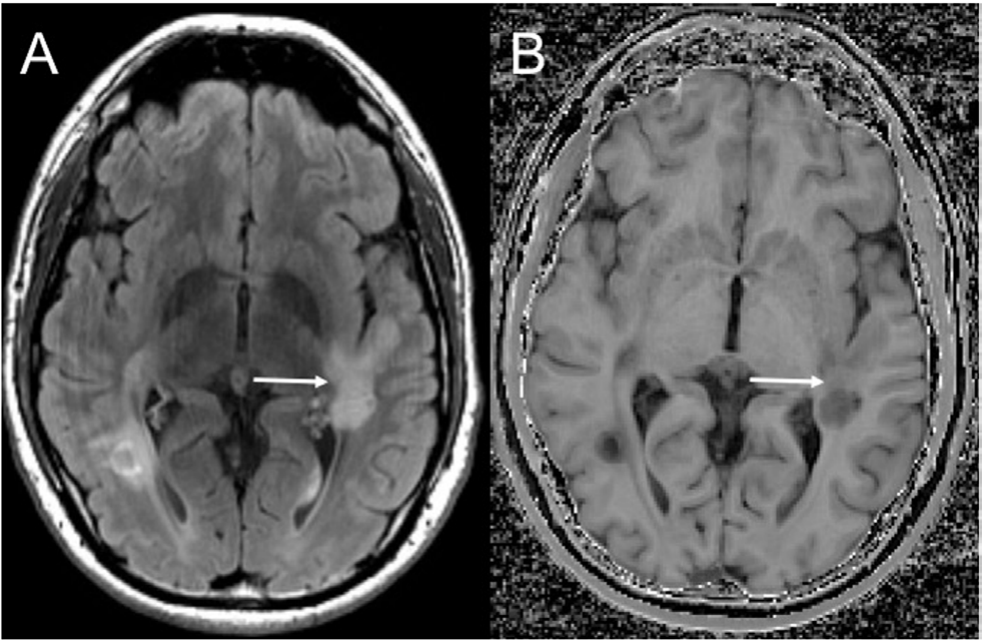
Magnetization transfer imaging. Axial FLAIR (A) demonstrating a large demyelinating lesion in the white matter posterior to the Sylvian fissure. Magnetization transfer image (B) demonstrates a band of normal white matter signal intensity across the mid aspect of the lesion (solid arrow) compatible with partial remyelination.
